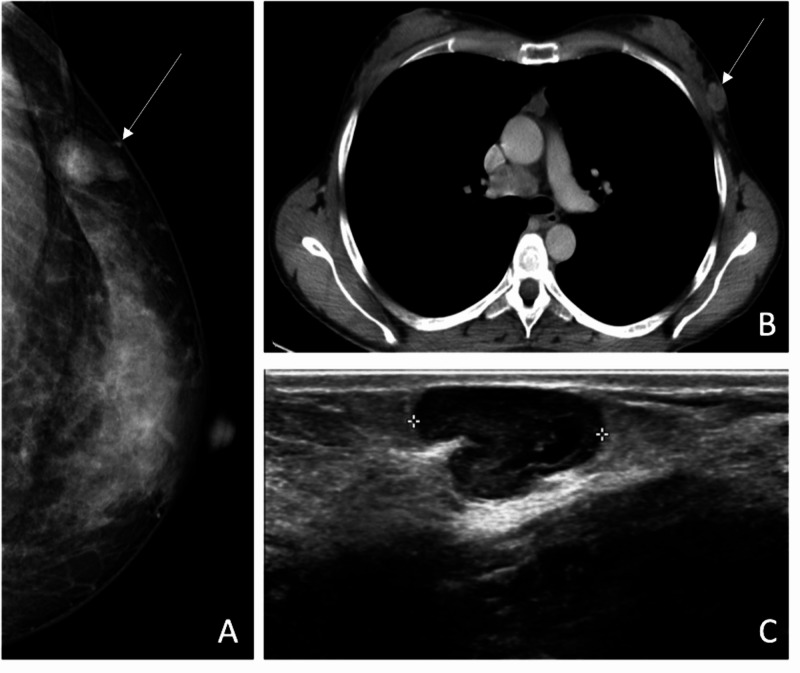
Patient with prior melanoma of the back. (A) Mammography demonstrates a round, hyperdense, circumscribed lesion, which is solid on CT scan (B). (C) Same lesion appears hypoechoic and lobulated on ultrasound.
